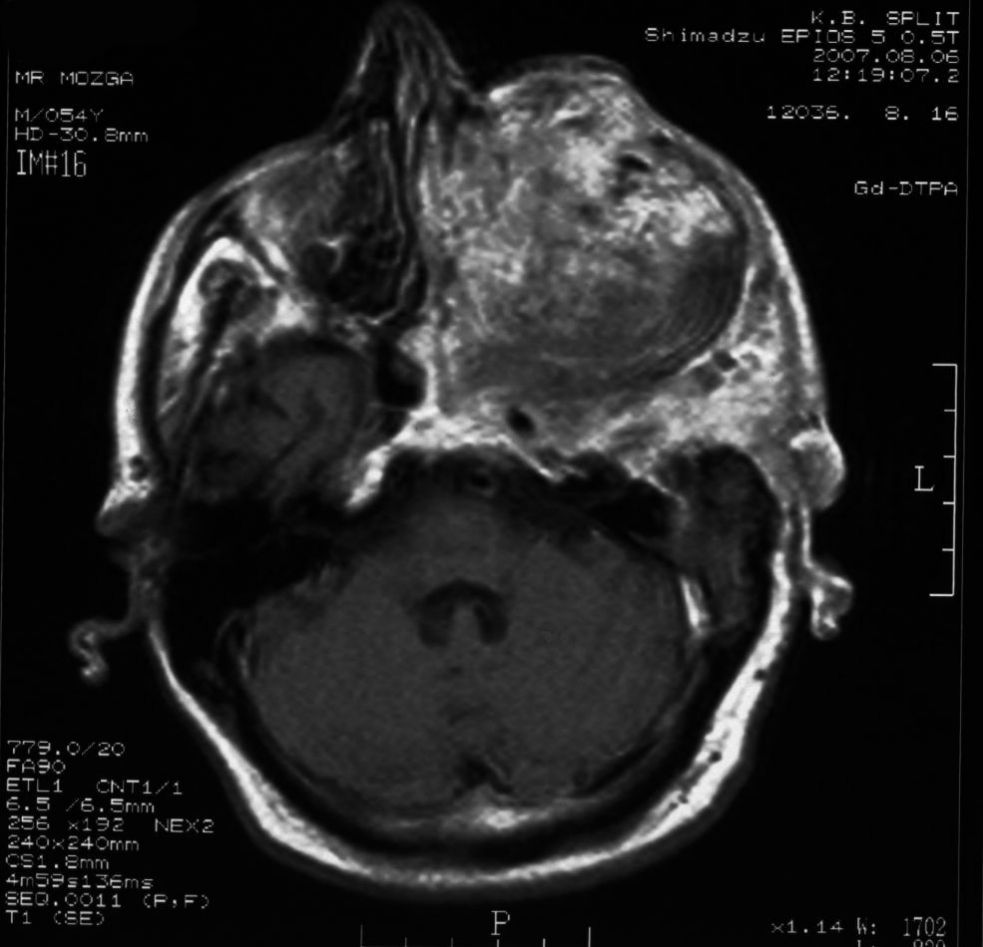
Axial T1-weighted contrast enhanced MRI image demonstrates extra cranial portion of the tumor extended to the sphenoid and ethmoid sinuses, nasal cavity, and orbit.
